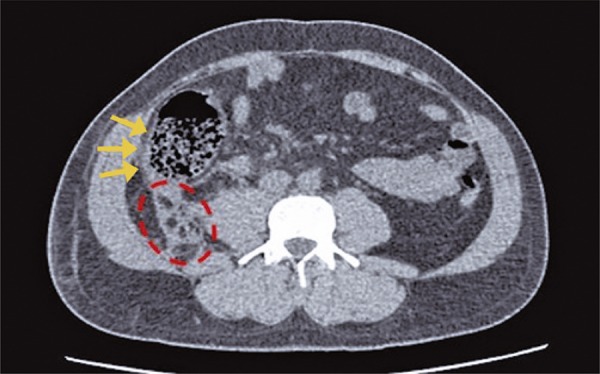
Axial computed tomography showing hemoperitoneum (circle) and right colon parietal thickening (arrows)
